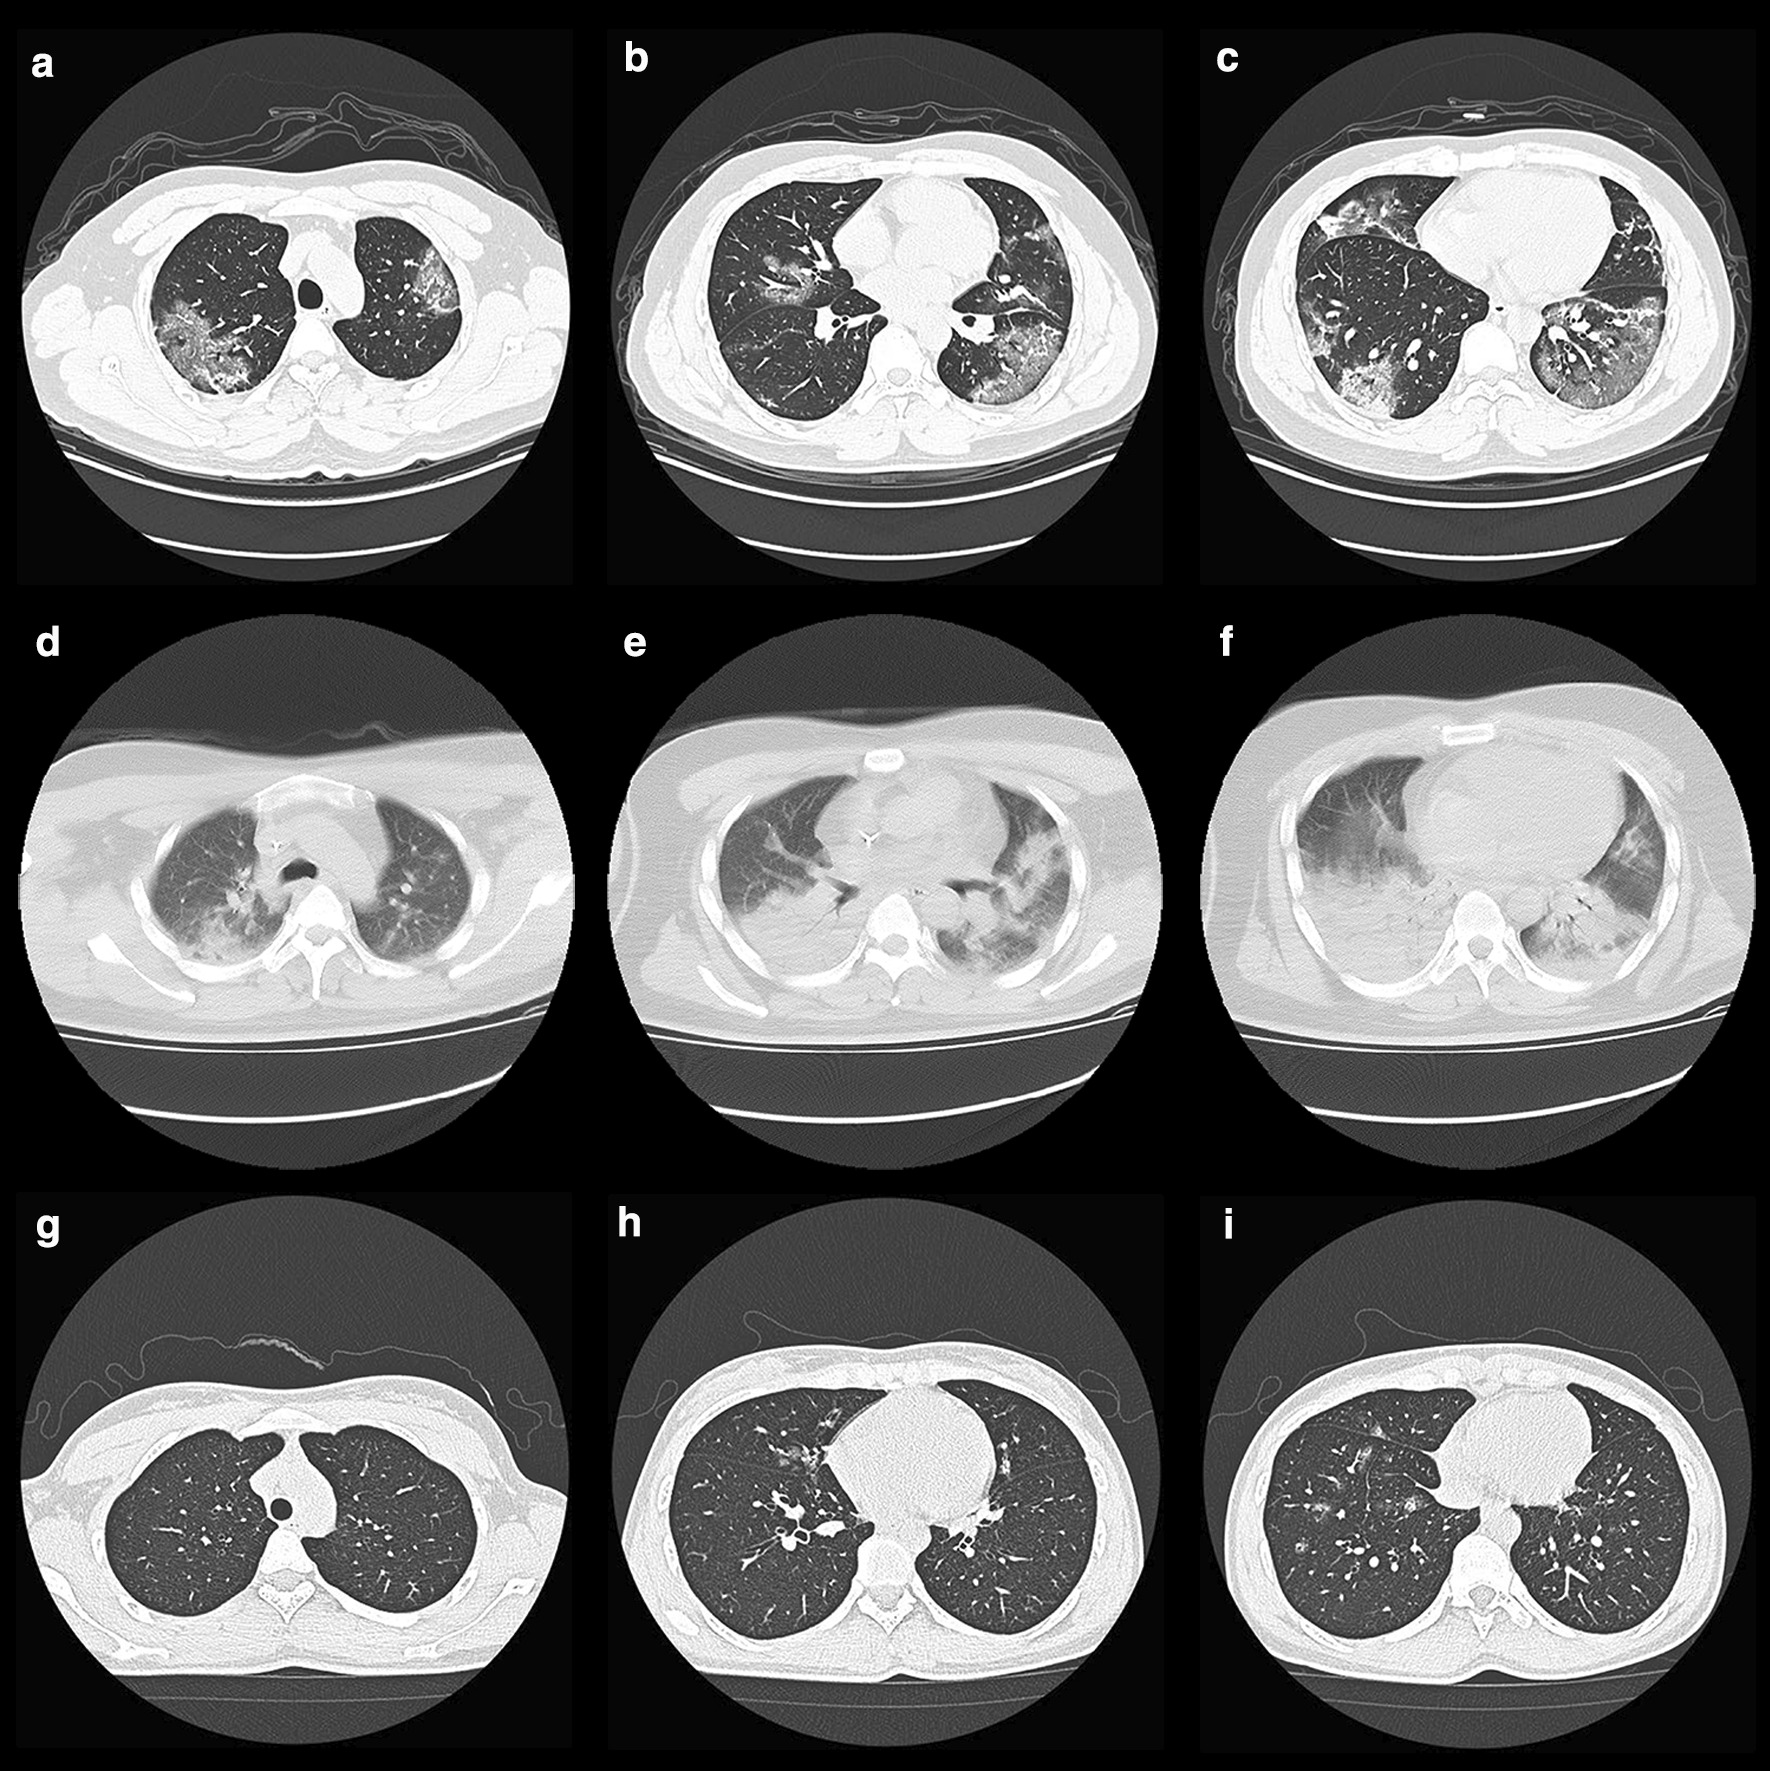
a–c Chest CT Images of a 36-year-old man with COVID-19 on admission, showed multiple ground-glass densification shadows with multiple diffusions in both lungs, mainly distributed under the pleura. d–f Chest CT Images of a 32-year-old man infected with H7N9 on admission, showed multilobar patchy consolidation and diffuse alveolar opacities. g–i Chest CT Images of a 29-year-old woman infected with H1N1 on admission, showed ground-glass opacity and small patchy shadows with diffused distribution in the right middle and lower lungs
